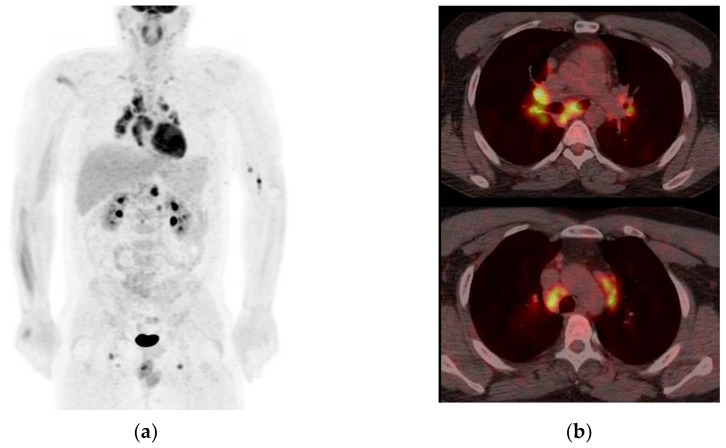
^18^F-FDG maximal intensity projection (a) and fused PET-CT axial slices showing multiple intense hypermetabolic mediastinal (b) and infra-mediastinal lymphadenopathies.
